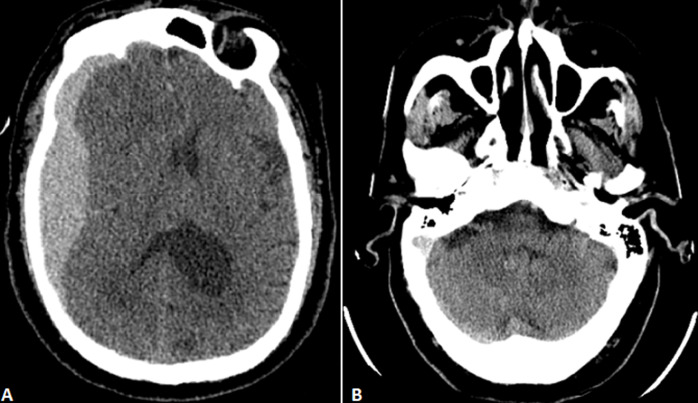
A) preoperative axial non-contrast CT scan showing a right-hemispheric acute subdural hematoma with right ventricular compression and midline-shift to the left; B) preoperative cerebellum view showing no abnormalities
